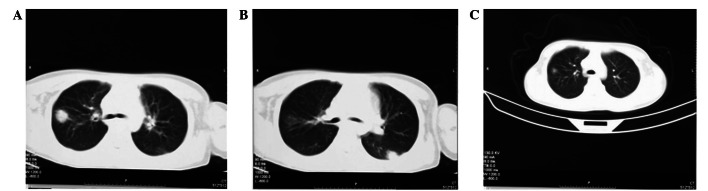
Pulmonary CT images prior to and following treatment. High density shadows were present in (A) the superior lobe dorsal segment of the right lung and (B) the inferior lobe of the left lung prior to treatment. (C) Following treatment, the original pulmonary hemorrhage was altered; it was clearly absorbed and reduced.
